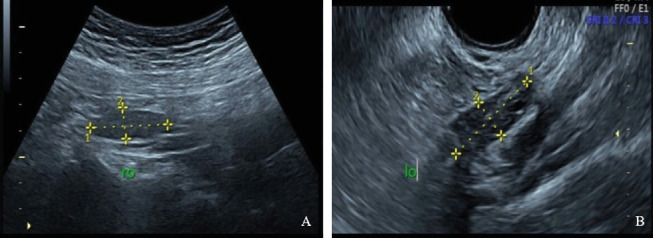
Combined transabdominal and transvaginal three-dimensional Doppler ultrasonography examination. (A) The right ovary is only detectable via transabdominal ultrasound. (B) The left ovary is visible through transvaginal ultrasound.
